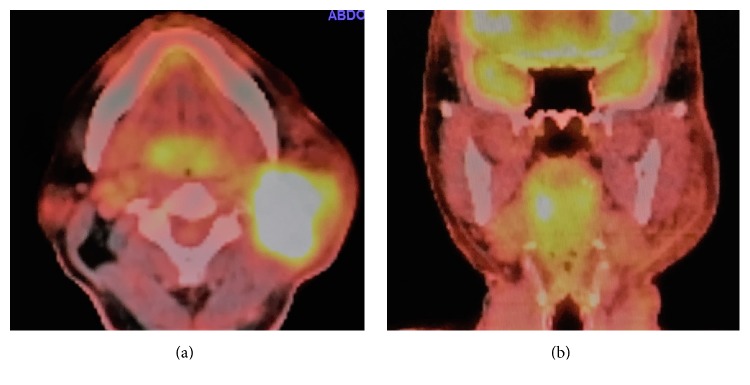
PET/CT scan ((a) axial view; (b) coronal view). A PET/CT showed a high accumulation of fluorodeoxyglucose (FDG) to the left neck mass, and there was a slight laterality of FDG accumulation in the tonsils with the right-side predominance. The tonsillar uptake of the FDG was within the physiological range, which was not conclusive for the diagnosis of the tonsillar primary lesion.
